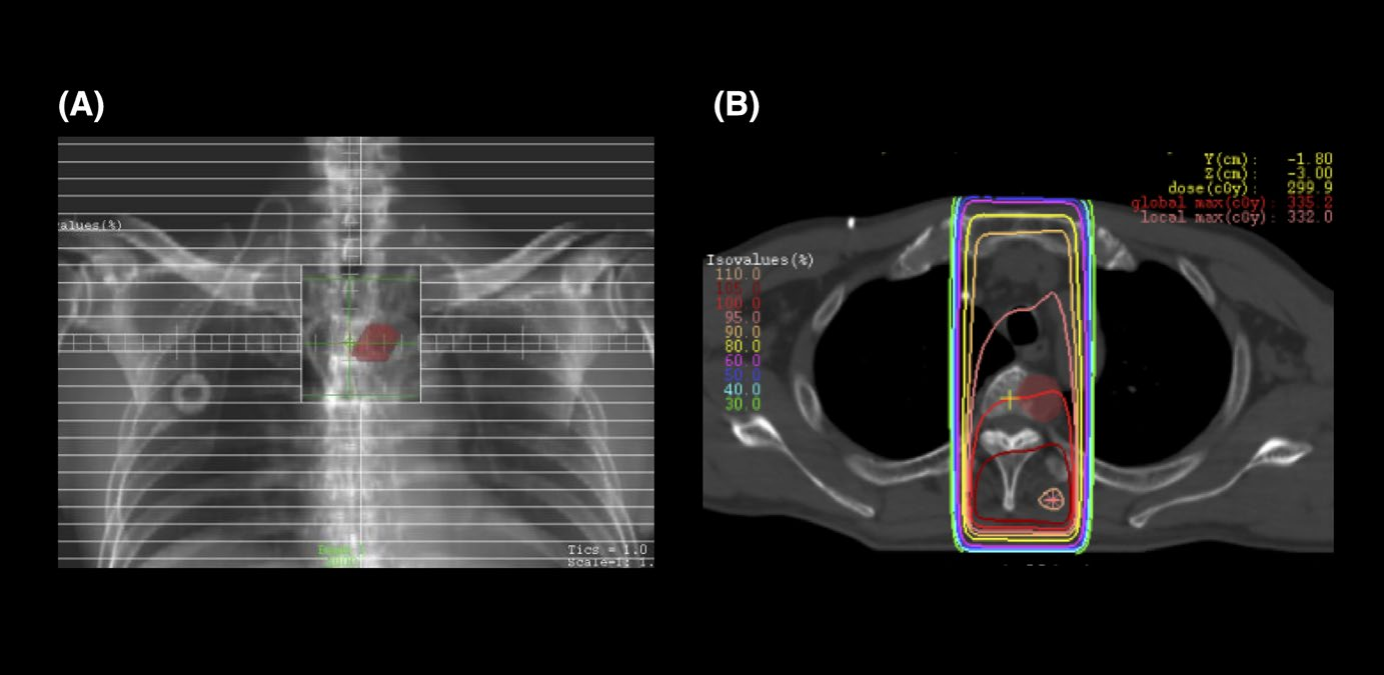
Palliative radiotherapy (RT) using 30 Gy delivered in 10 fractions for 2 wk was delivered to the third thoracic vertebra. Digital reconstructed radiography (A). Dose distribution in axial slice (B)
